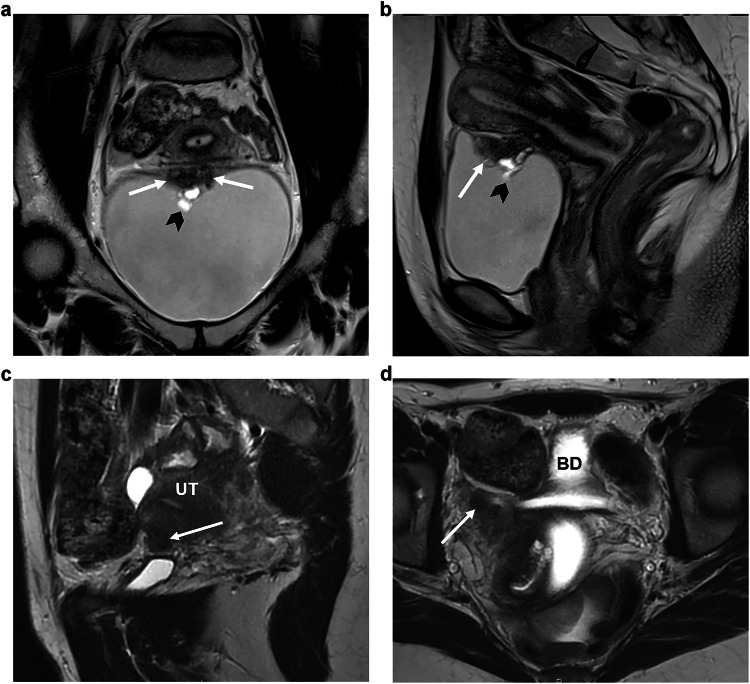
Bladder and round ligament endometriosis. a, b Bladder endometriosis in a 29-year-old woman with dysuria. The coronal (a) and sagittal (b) T2W MR images reveal a well-defined, low-signal-intensity nodule (arrows) in the bladder wall projecting into the lumen. The nodule exhibits heterogeneous content due to small cystic areas indicative of glandular components (arrowhead). c, d Endometriosis affecting the proximal third of the right round ligament in a 27-year-old woman with chronic pelvic pain. The sagittal (c) and axial (a) T2W MR images demonstrate low-signal intensity nodular thickening of the round ligament at its uterine insertion site (arrows). UT, uterus; BD, bladder
